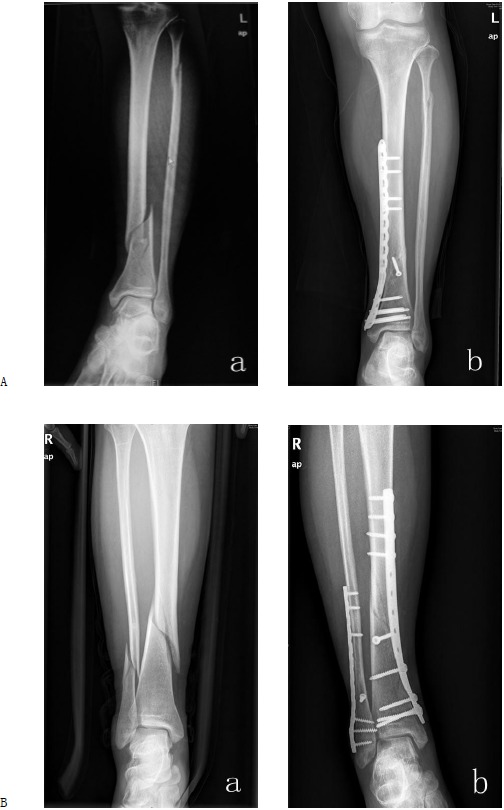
The X-ray images of typical plating cases. (A) a 47-year-old female with Type A1 left distal tibia fracture and proximal fibula fracture due to a traffic accident. a: The prooperative radiographs is shown; b: The fracture was treated with a locking plate (LCP). The fracture healed 4 moths after the operation. (B) a 44-year-old female with Type A1 right distal tibial fracture and fibula fracture due to a traffic accident. a: the preoperative radiographs is show; b: The fractures were treated with two non-locking plates (DCP) for the tibial and fibula. The fractures healed 3 months after the operation.
